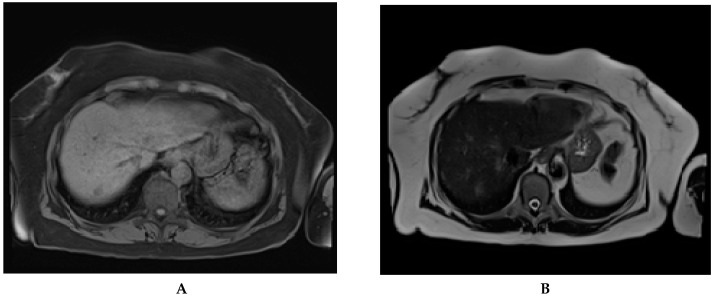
Abbreviated magnetic resonance imaging done for HCC surveillance in a 74 years old cirrhotic patient. A newly discovered nodule, in segment 7, is to be seen on the T1 vibe fs tra sequence (A) and T2 haste tra sequence (B). The nodule was later confirmed and classified as LI-RADS 5 by CEUS.
